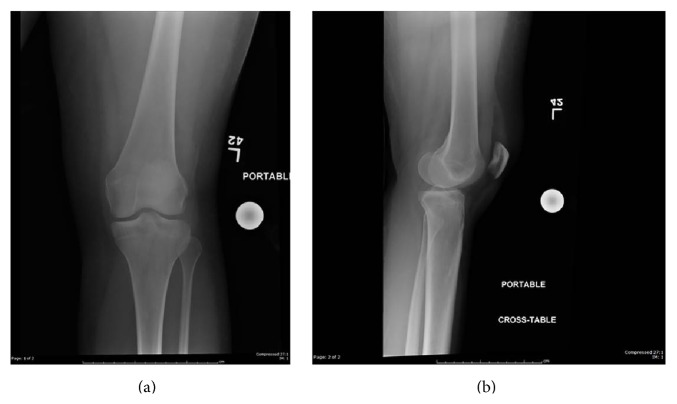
AP and lateral plain radiographs of the left knee showing a nondisplaced, vertically oriented fracture of the lateral tibial condyle.
